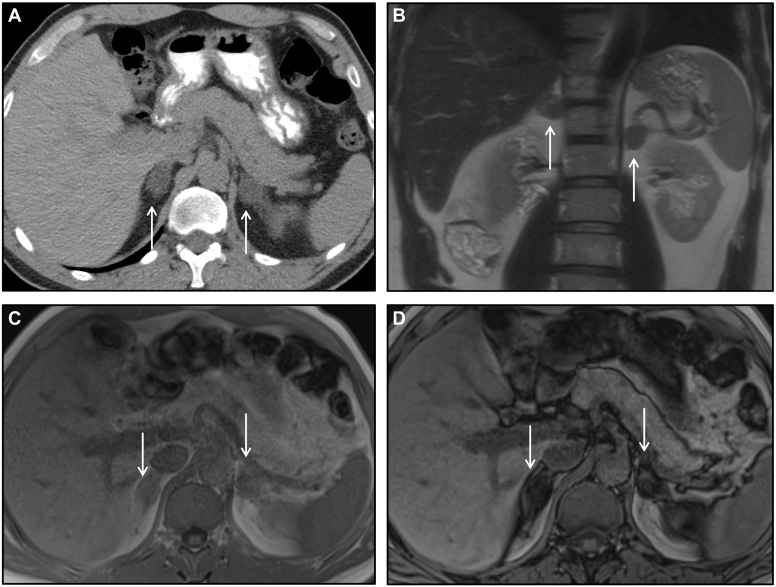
Bilateral adrenal adenomas. (*A*) Axial noncontrast CT image demonstrates bilateral adrenal nodules (arrows) with homogenous low density <10 HU, highly specific for benign lipid-rich adenoma. (*B*) Axial T2 -weighted image in the same patient shows homogenous low-signal intensity signal of the adrenal lesions (arrows) comparable to the liver. (*C*, *D*) Chemical shift MRI images (C in-phase, D opposed-phase) showing signal drop on opposed-phase images (arrows in D) where the lesions are now much darker than in C, consistent with intralesional fat, classic of lipid-rich adenomas. *CT* = computed tomography; *MRI* = magnetic resonance imaging.
